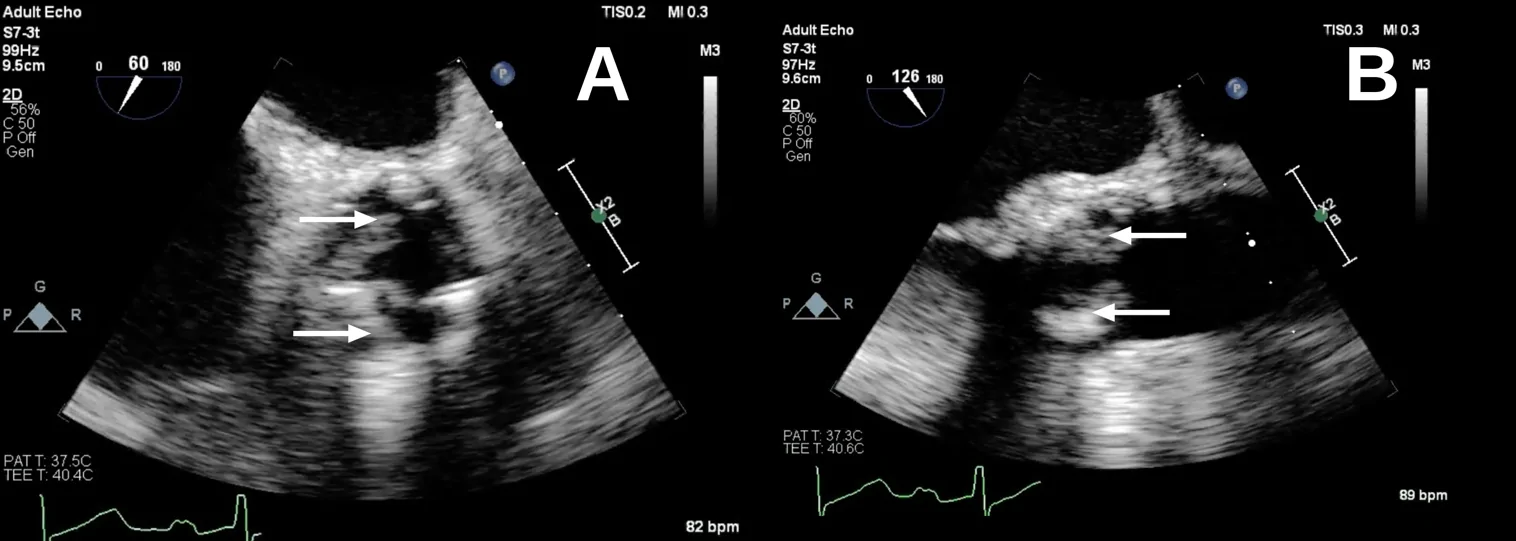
Transesophageal echocardiography in December 2021. (A) View obtained at a multiplane angle of 60°; (B) view obtained at 126°. Arrows indicate abnormally thickened bioprosthetic aortic valve leaflets without definitive vegetations or periannular complications.
